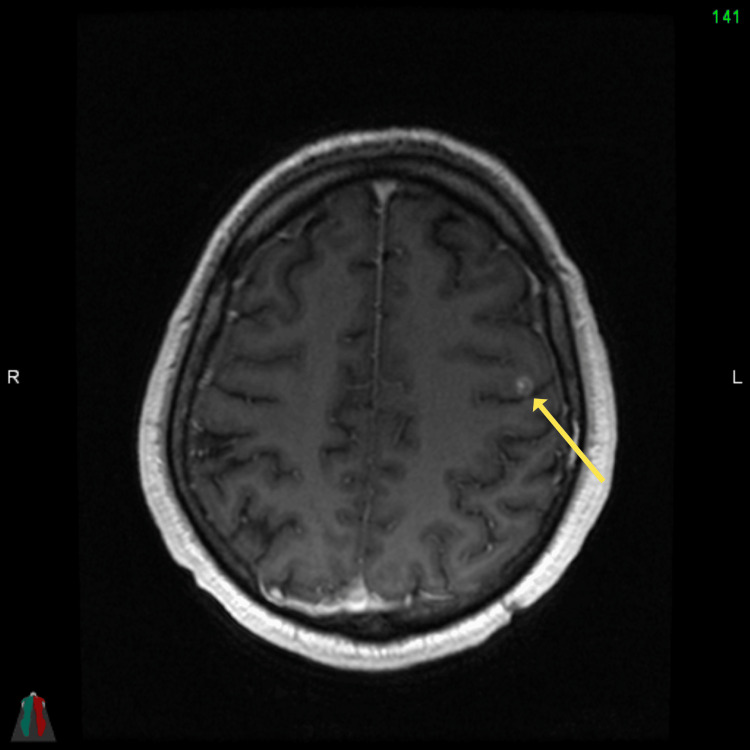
Axial head MRI T1 FSPGR post-contrast demonstrating left frontal lobe nodule measuring 6 mm in keeping with metastatic deposit FSPGR: fast spoiled gradient-echo
